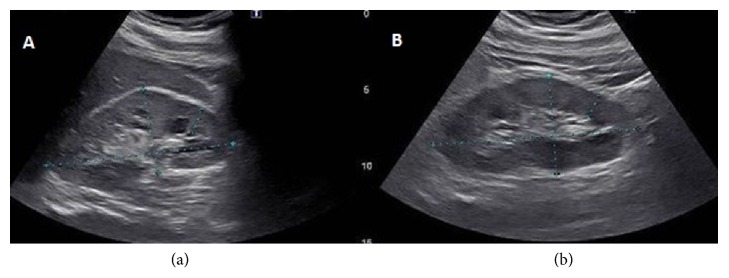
Renal ultrasound. (a) Sagittal section of the right kidney demonstrating increased echogenicity. The right kidney measures 12.3 x 5.5 x 5.9 centimeters. (b) Sagittal section of the left kidney demonstrating increased echogenicity (blue line). The left kidney measures 13.4 x 6.4 x 5.2 centimeters.
